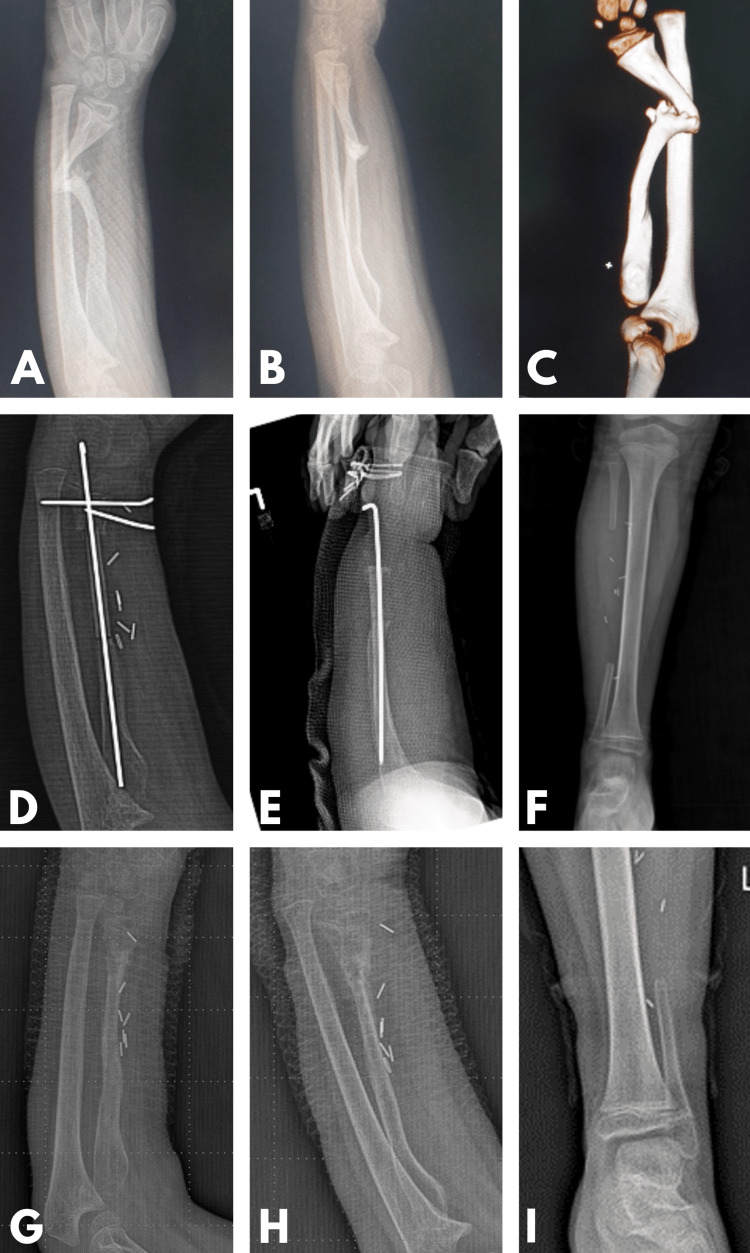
Evaluations and interventions when the patient reached six years of age A, B, C. Initial evaluation X-ray and three-dimensional (3D) computed tomography images. D, E, F. X-ray images of forearm and cruris after vascularized fibula operation. G, H, I. X-ray images of forearm and cruris six months after vascularized fibula operation.
